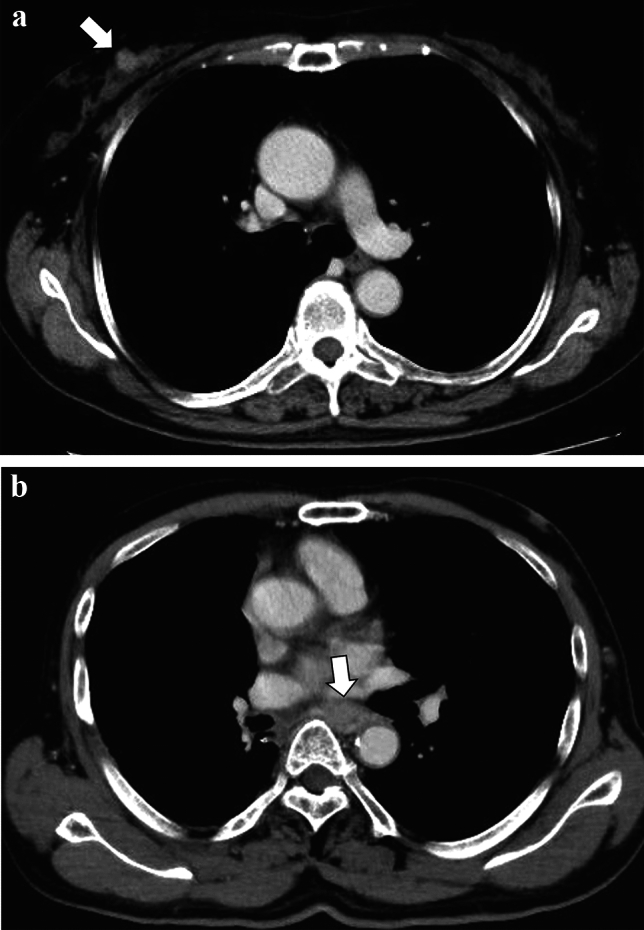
CT images of a 70-year-old female patient with a right breast carcinoma (a) and a 78-year-old male patient with esophageal carcinoma (b). Both carcinomas (white arrows in (a) and (b)) were detected by the fine-tuned large multimodality model and reader 1, while reader 2 missed those lesions
